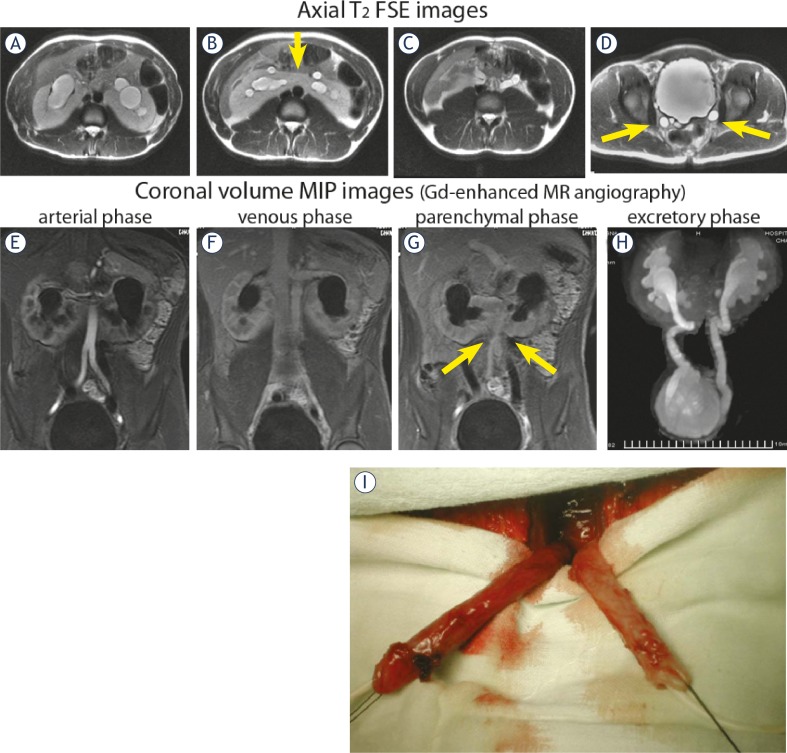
MR imaging of horseshoe kidney in 12-year-old boy. A-D. Axial T2 FSE images – clearly dilated pyelocalyceal system and ureters in horseshoe kidney. E. Coronal volume MIP image from arterial phase of 3D Gd-enhanced MR angiography – the main left and right renal arteries extending from the anterior aspect of the aorta. F. Coronal volume MIP image from venous phase – both renal veins in their expected locations. G. Coronal volume MIP image from parenchymal phase – lower poles of the kidneys without any parenchymal abnormalities. H. Coronal volume MIP image from excretory phase – marked dilatation of both pyelocalyceal systems and ureters. I. Intraoperative findings prove the diagnosis of bilateral megaureters in horseshoe kidney with dysplastic changes in their distal thirds.
